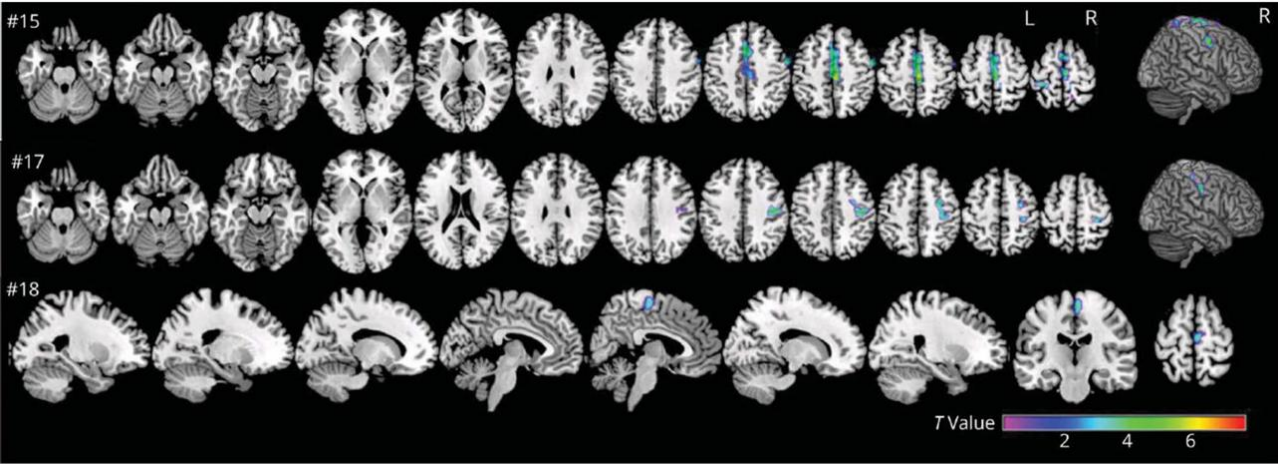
Brain ^18^F-FDG PET/CT imaging in patients with LAS. The colour scale (T-value) shows regions with glucose hypometabolism in patients with LAS versus 55 healthy controls, on a projection onto T1-weighted MR images. Significant hypometabolism is found in the motor and pericentral cortical areas: pre-central gyrus (upper and middle parts), post-central gyrus (upper part), paracentral lobule (upper part) and supplementary motor area (upper and lower parts). L, left; R, right. This figure is published in Vellieux *et al.*^8^ and reused with permission (Copyright Wolters Kluwer Health Inc.).
